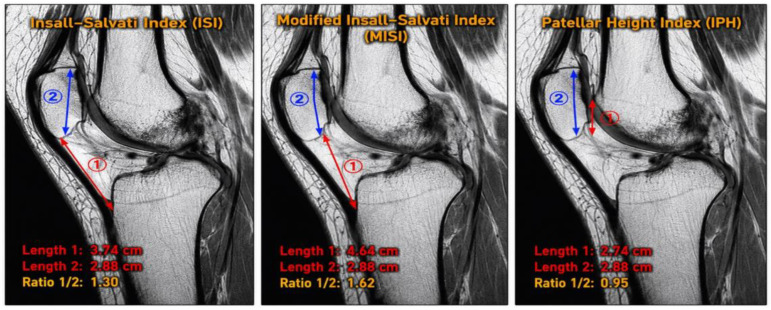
MRI assessment of patellar height and engagement.
